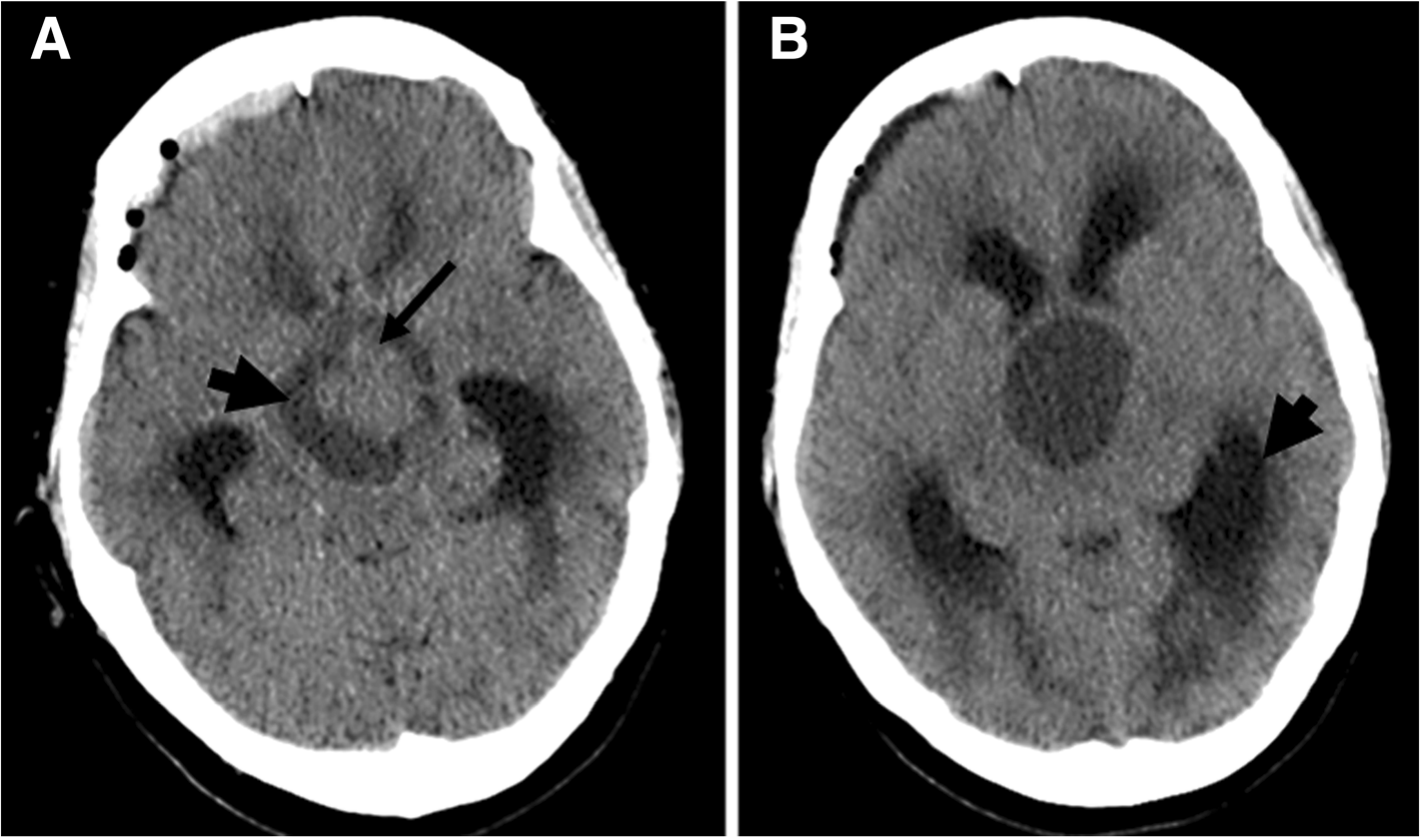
Computed tomography scan performed in Emergency Department. a Massive lesion with a solid component (*thin arrow*) and a cystic/necrotic component (*thick arrow*). b Obstructive hydrocephalus and dilatation of the lateral ventricles, especially on the left side (*thick arrow*)
